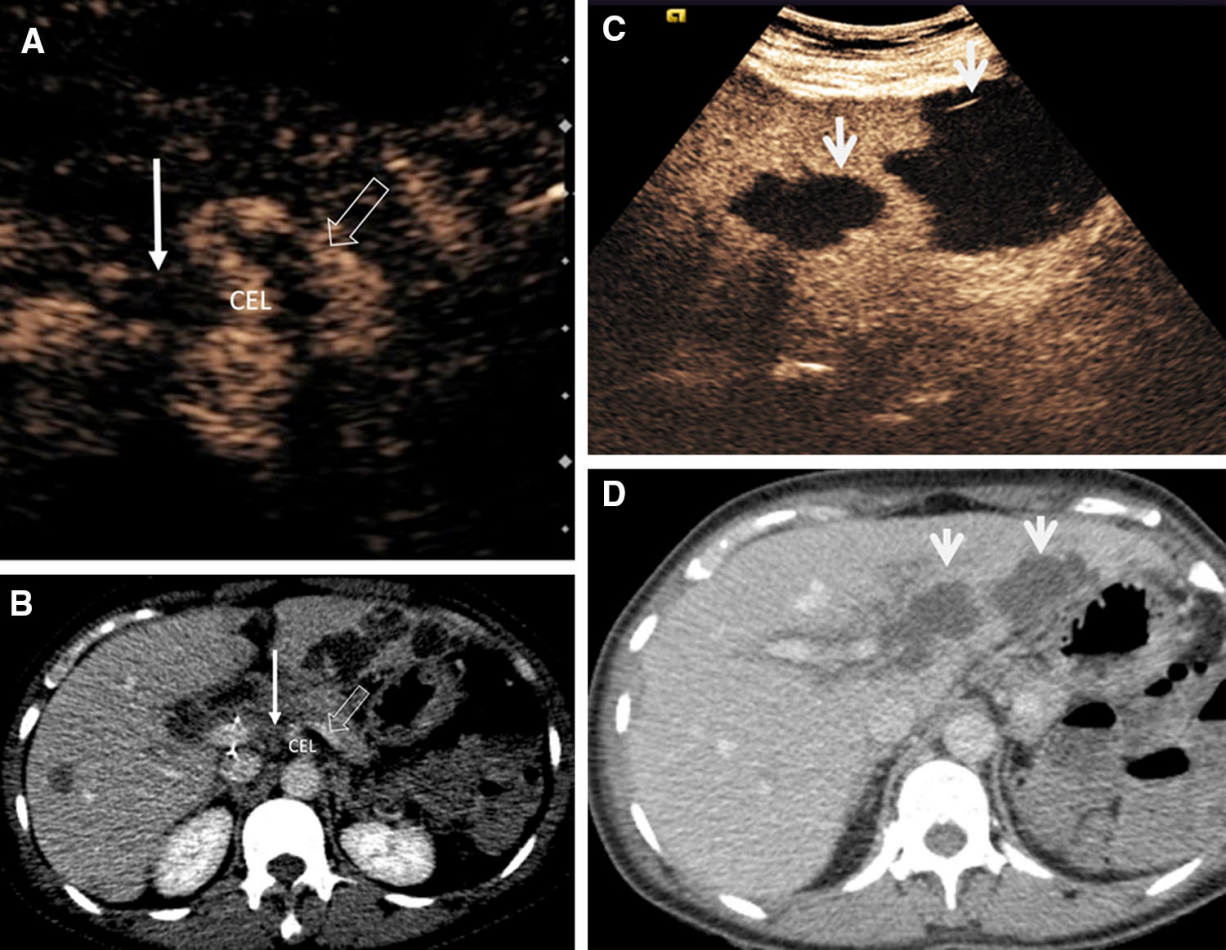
A 60-year-old female developed hepatic artery thrombosis post-liver transplantation. The CEUS (A) and axial CECT (B) comparison images demonstrate proximal hepatic artery thrombosis (solid arrow) with lack of microbubble ultrasound contrast or iodinated contrast vascular enhancement. The coeliac trunk is labeled as CEL and the splenic artery is indicated by open arrows. There is widespread resultant geographical areas of hepatic infarction present on the CECT and CEUS images (arrows, C and D)
